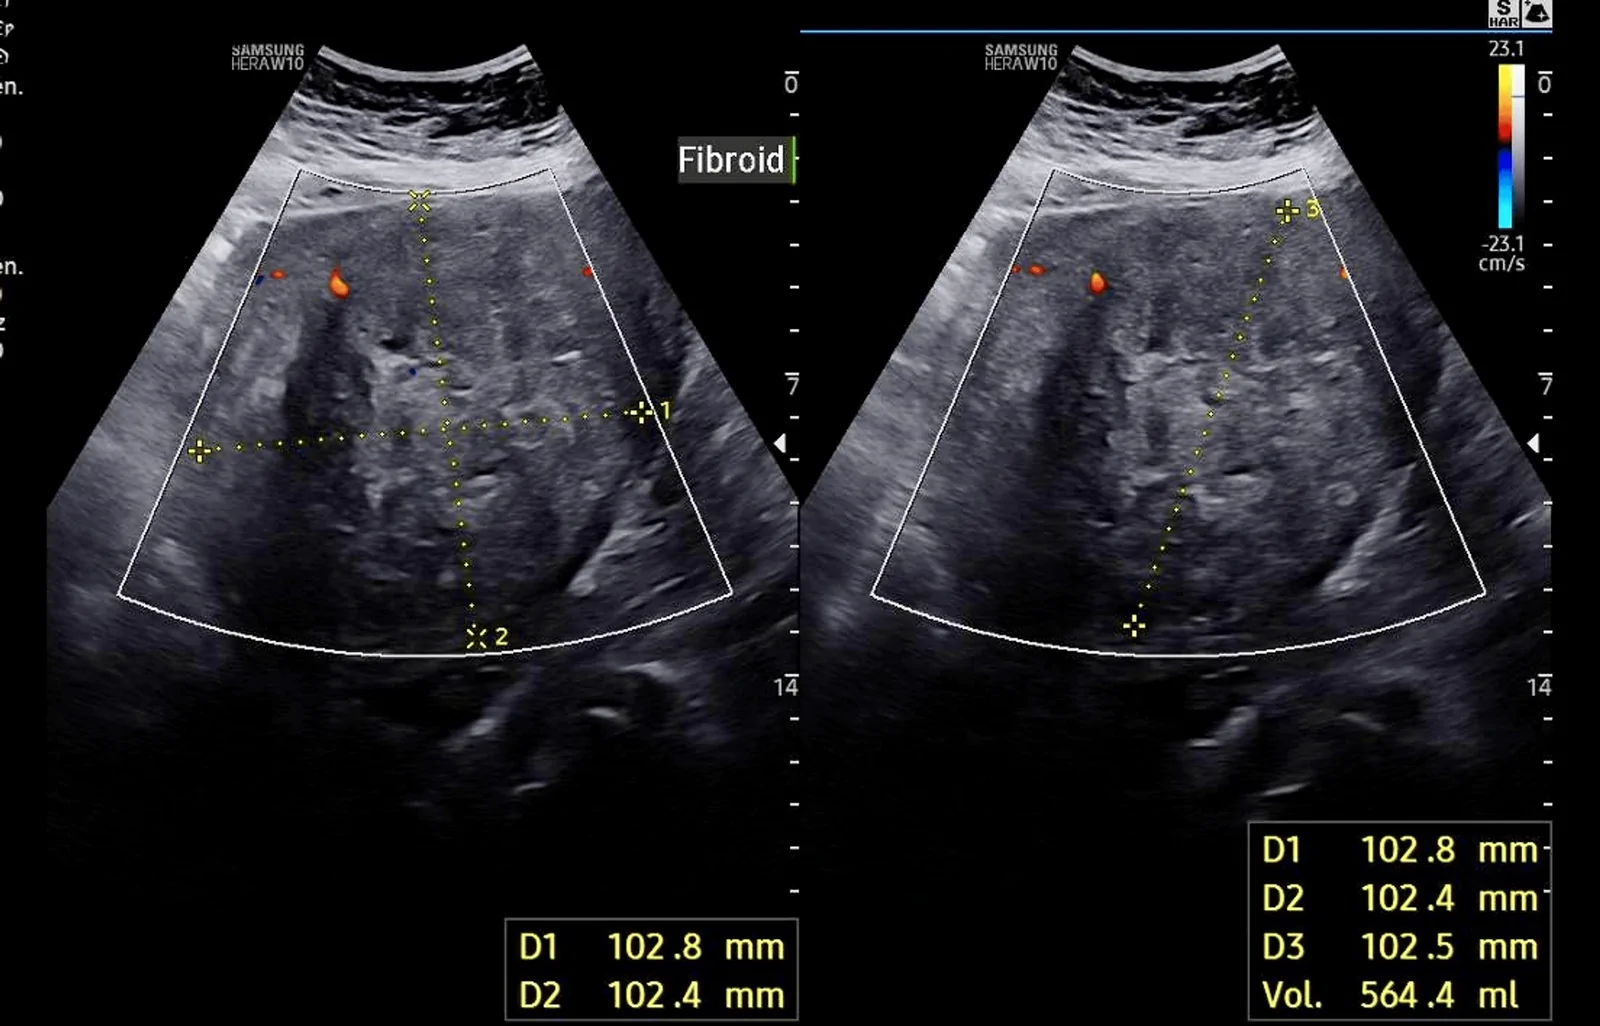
Early pregnancy ultrasound demonstrating a large uterine fibroid measuring approximately 102.8 × 102.4 × 102.5 mm.
